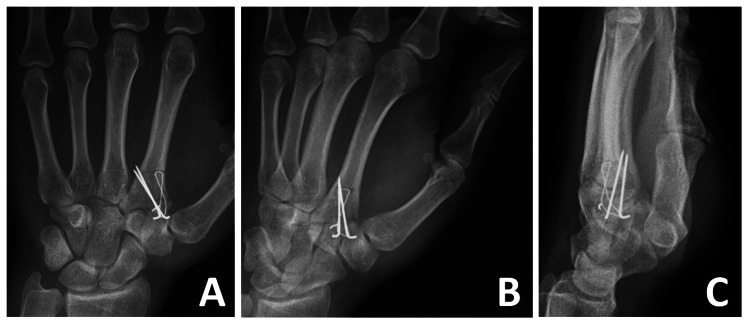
Postoperative (A) posteroanterior, (B) oblique, and (C) lateral hand radiographs
